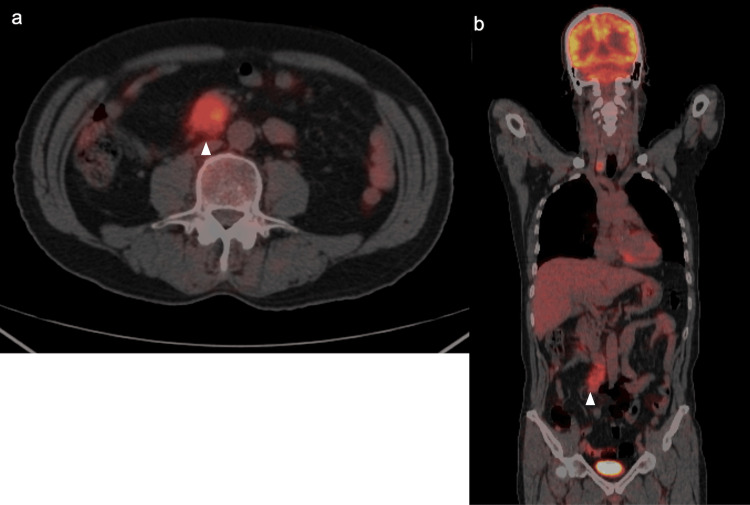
FDG-PET CT scan FDG-PET CT revealed FDG uptake with an SUVmax of 5.1 in the same lesion (a: axial view; b: coronal view). Arrows indicate tumor locations. FDG-PET, fluorodeoxyglucose positron emission tomography; CT, computed tomography
